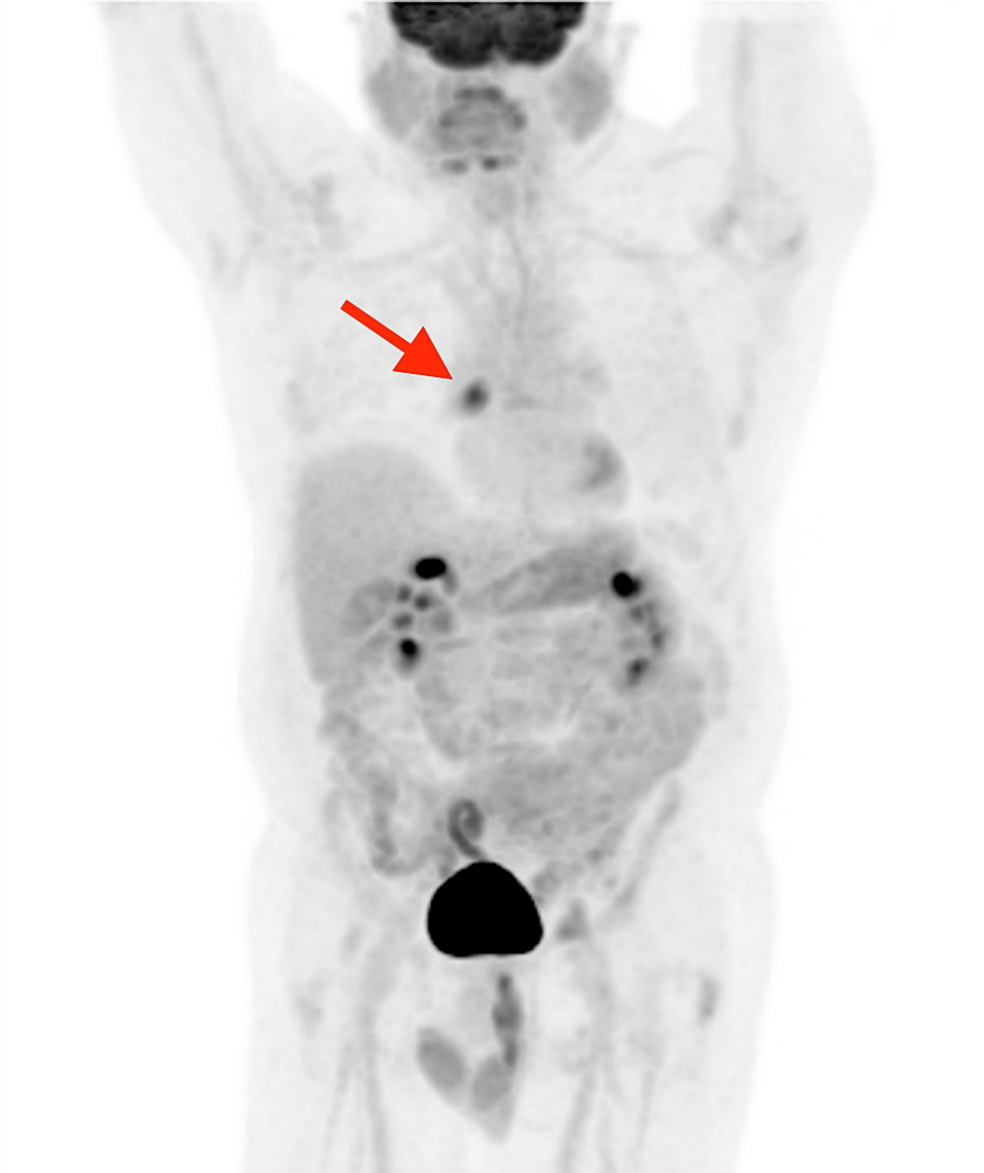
A coronal maximum intensity projection image of the FDG PET-CT revealing a hypermetabolic focus in the right hemithorax (red arrow).
